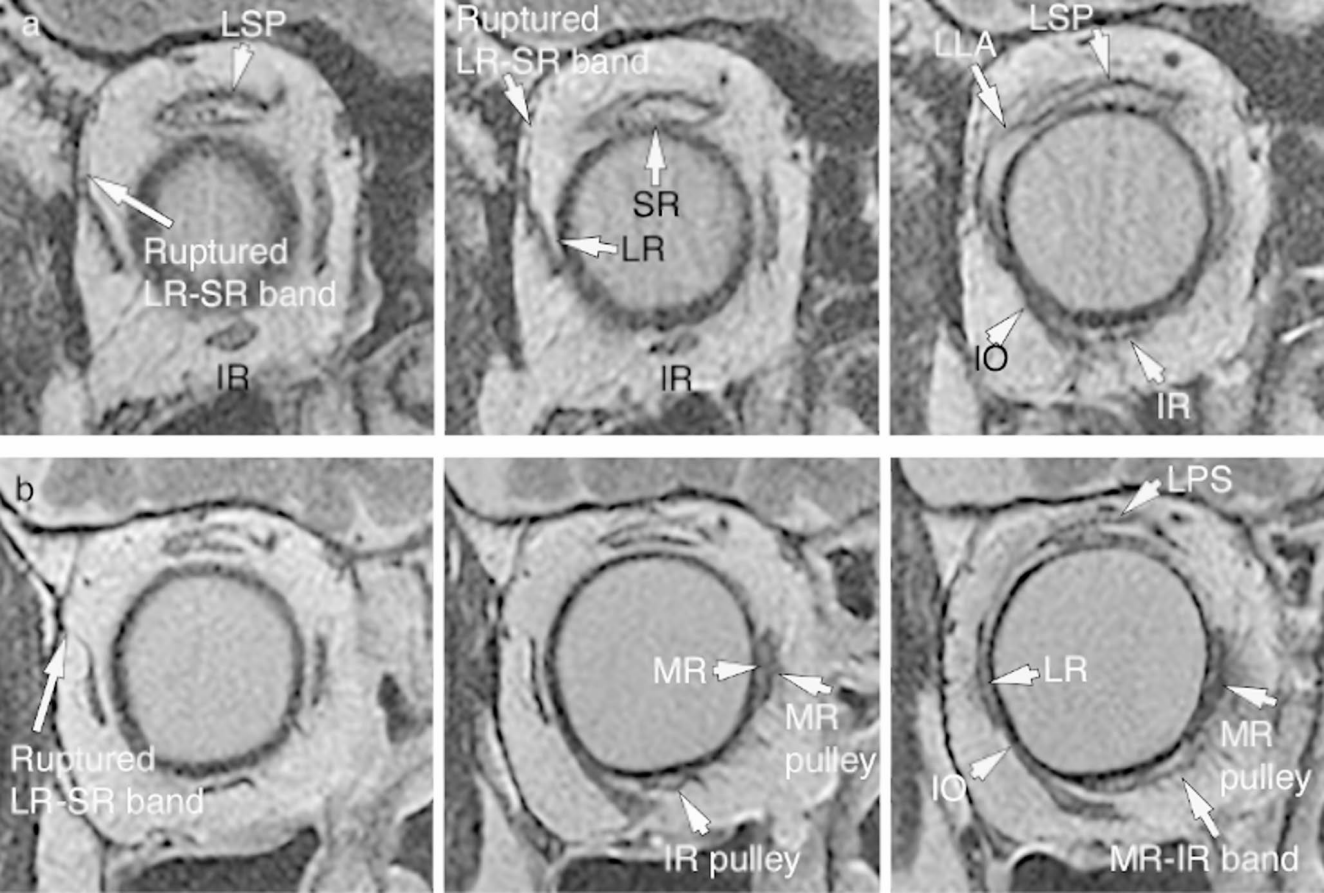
T2-weighted quasi-coronal images of patients with sagging eye syndrome. a Left orbit of patient number 3 (80-year-old female). The image in the left column was 2 mm anterior to the globe/optic nerve junction, and that in the center column was 4 mm anterior. The tilt of the LR with a ruptured LR-SR band (28°) was greater than that in patient number 4 (13°). The pulley positions of the MR (–2.5 mm) and IR (–14.5 mm) were inferior to those of patient number 4 (–0.1 mm and –9.8 mm, respectively). The LLA bulged superotemporally in the image of the right column (6 mm anterior to the globe/optic nerve junction). b Right orbit of patient number 4 (75-year-old female). A ruptured LR-SR band was observed in the left column (4 mm anterior to the globe-optic nerve junction). In the center (6 mm anterior) and right column (8 mm anterior), the IR pulley orbital, MR pulley orbital, and MR-IR band had low signal intensities. The MR and IR, which had higher signal intensities than the pulley, were surrounded by a lower signal pulley. Compared with patient number 3, no inferior shifts were observed in the MR and IR pulleys. The connection between the global side of the LR and the orbital side of the IO was observed. *LR* lateral rectus, *MR* medial rectus, *SR* superior rectus, *IR* inferior rectus, *LLA* lateral levator aponeurosis, *LPS* levator palpebrae superioris, *IO* inferior oblique
